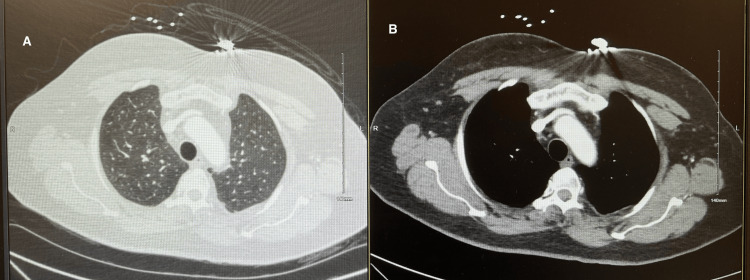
CT angiography of the chest suggesting no evidence of pulmonary embolism (A) CT angiography of the chest in the lung window suggesting no evidence of pulmonary embolism. (B) CT angiography of the chest in the mediastinal window suggesting no evidence of pulmonary embolism
